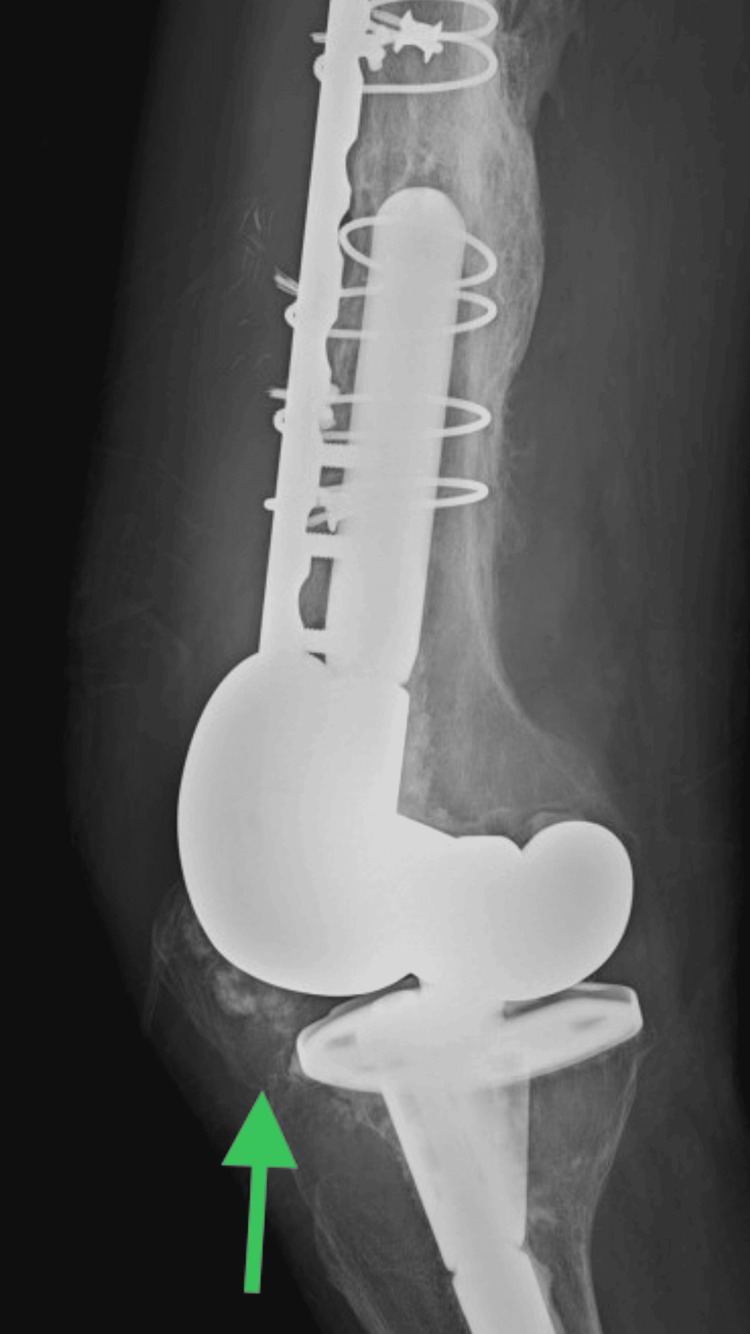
Right knee X-ray The right knee X-ray revealed no joint effusion and suboptimal prepatellar soft tissue edema (Green arrow). X-ray is not very significant.
